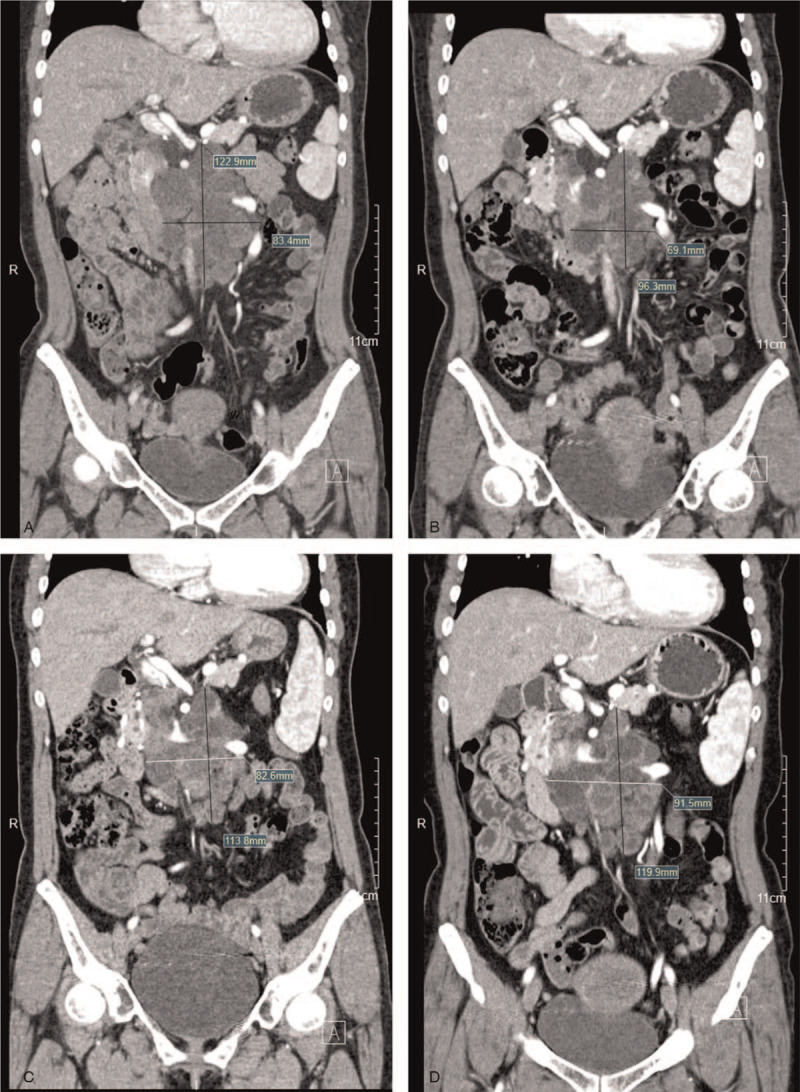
Coronal contrast-enhanced CT images at 4 follow-up examinations: January 24, 2018 (A); May 14, 2018 (B); April 22, 2019 (C); December 10, 2019 (D). (A-B) The mass was considerably reduced in size. (B-D) The mass gradually became slightly enlarged. CT = computed tomography.
